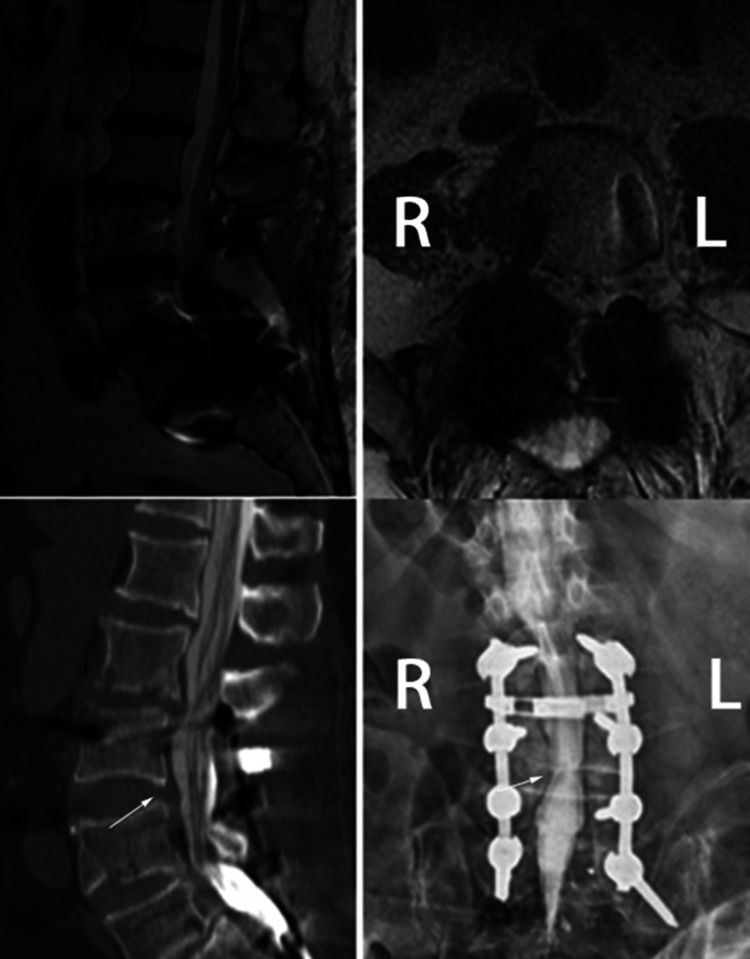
The patient had previously undergone a stabilization surgery. On the new admission of the patient, there was a complaint of pain in both legs. Myelogram and CT myelogram after MRI revealed dural sac compression at the L3-L4 level.
